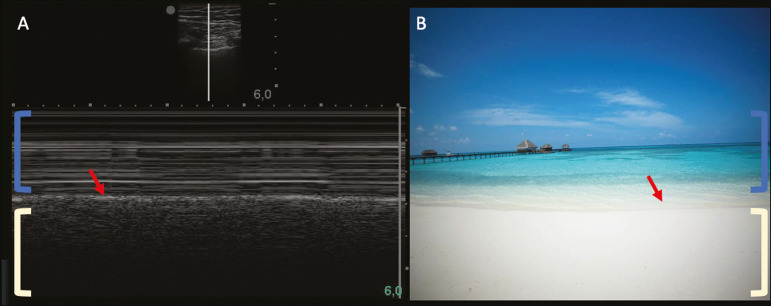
B-mode chest ultrasound shows the representation of two dimensions in the longitudinal section. A: Seashore sign. B: Analogy to “beach sand” and “sea wave” (seashore sign). Above the pleural line (arrows), the superficial tissues without movement are visualized in the form of lines parallel to the pleura, similar to “sea waves” (blue brackets). Below the pleural line, an artifactual image of the pleural sliding similar to “beach sand” (white brackets) is obtained. These findings are known as the “seashore sign”.
